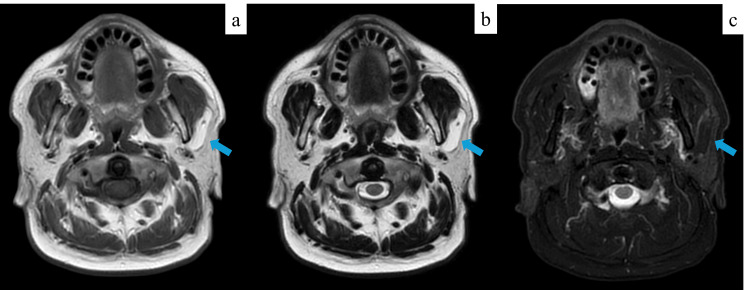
Preoperative magnetic resonance imaging. (a) T1-weighted and (b) T2-weighted images showing a well-defined 3 cm mass with high signal intensity located between the parotid gland and masseter muscle.
